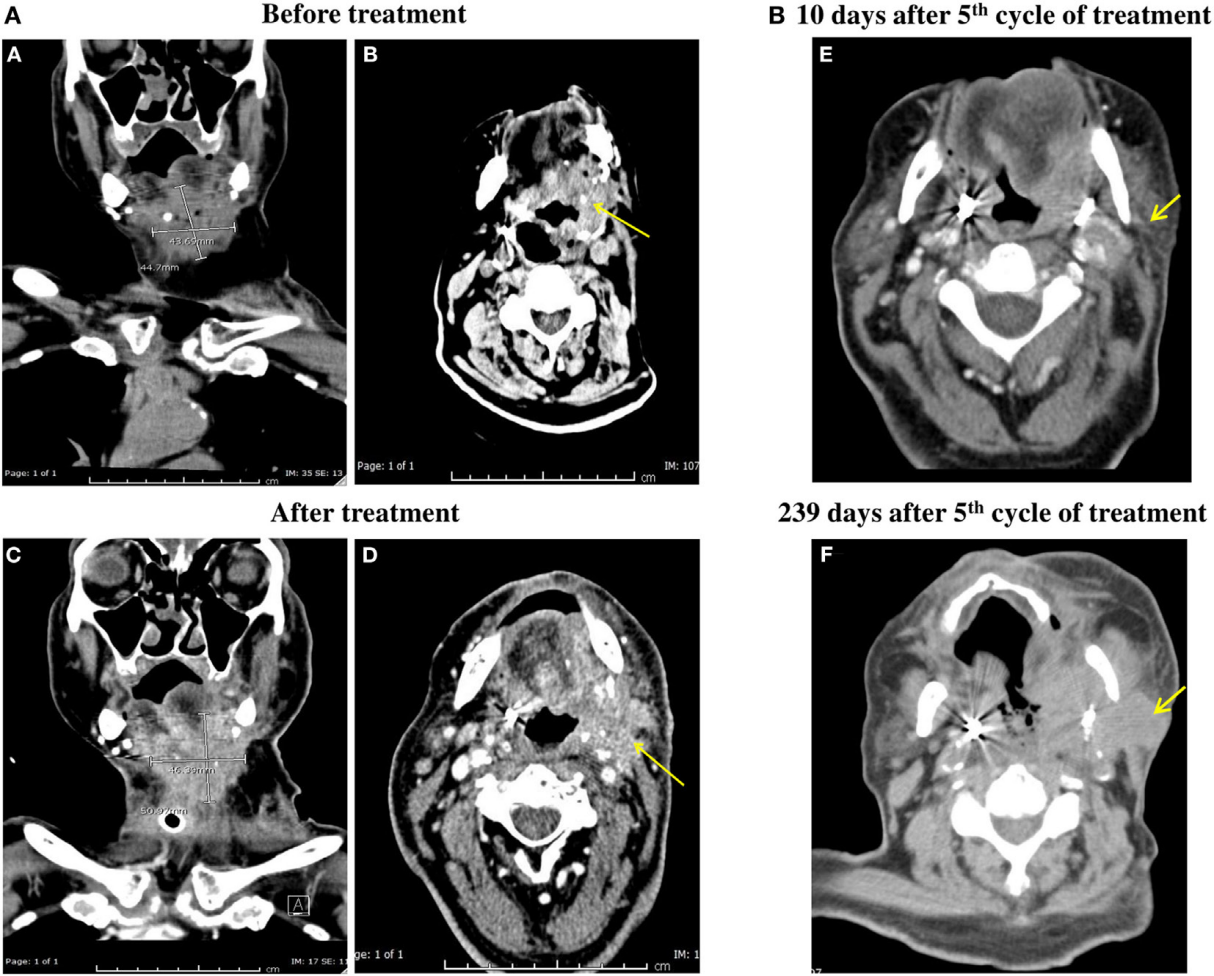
(A) CT scan of the patient neck with IV contrast. Irregular infiltrative mass in the left side of the neck adjacent to the base of the tongue, invading the oropharynx and extending caudally to supraglottic and glottic larynx was shown both before and after the fifth cycle of anti-programmed cell death protein-1 (PD-1) treatment [(B,D) respectively]. It shows mild increase in size measuring about 5.1 cm × 4.6 cm 10 days after the fifth cycle (C) compared to 4.5 cm × 4.3 cm before anti-PD-1 treatment (A). (B) PET CT carried out at day 239 after fifth cycle (7 months, 25 days) of anti-PD-1 treatment showing progression of the disease (F) compared tp PET CT obtained at 10 days after the fifth cycle (E).
